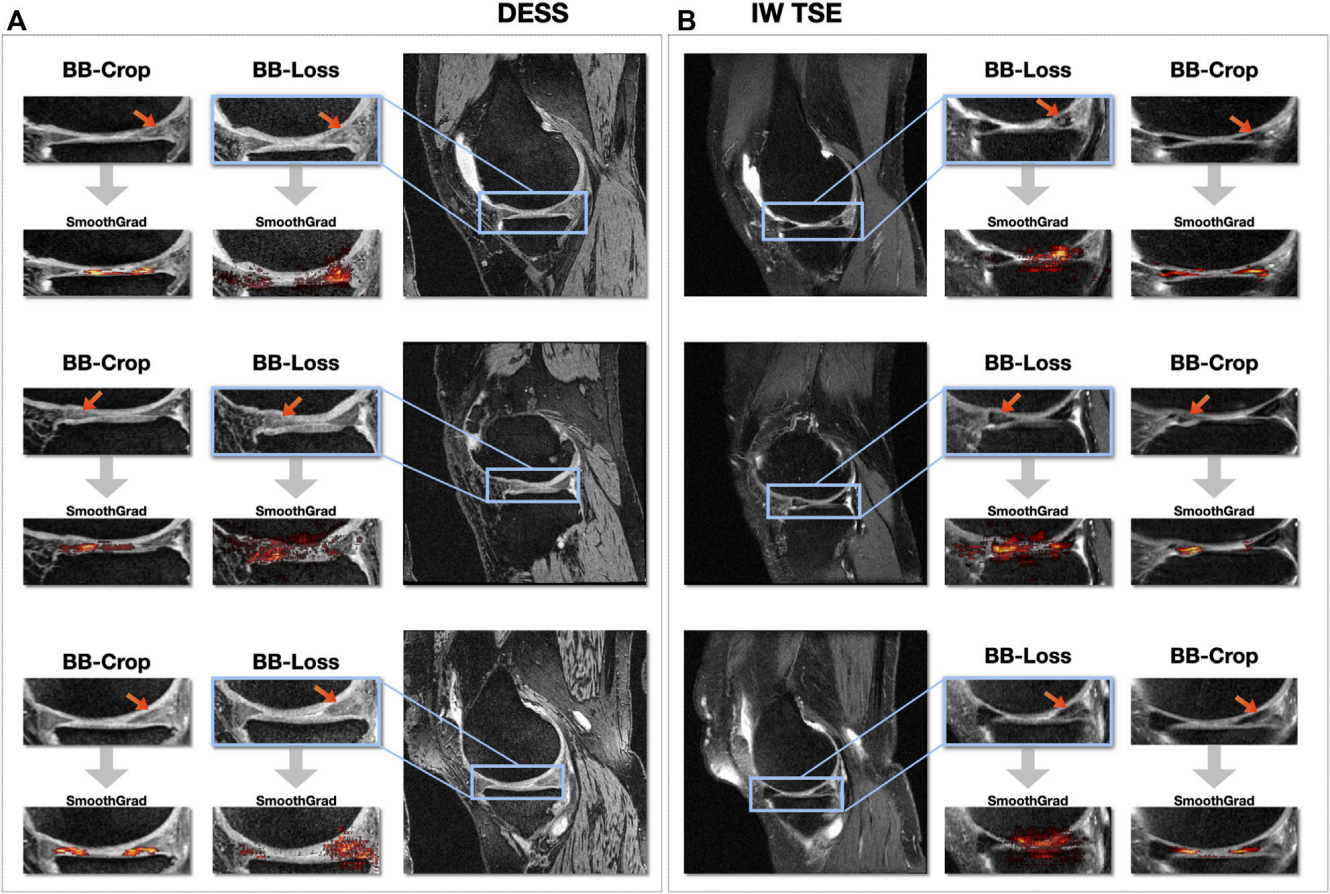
SmoothGrad saliency maps overlaid over DESS MRI data (A) and IW TSE MRI data (B).
